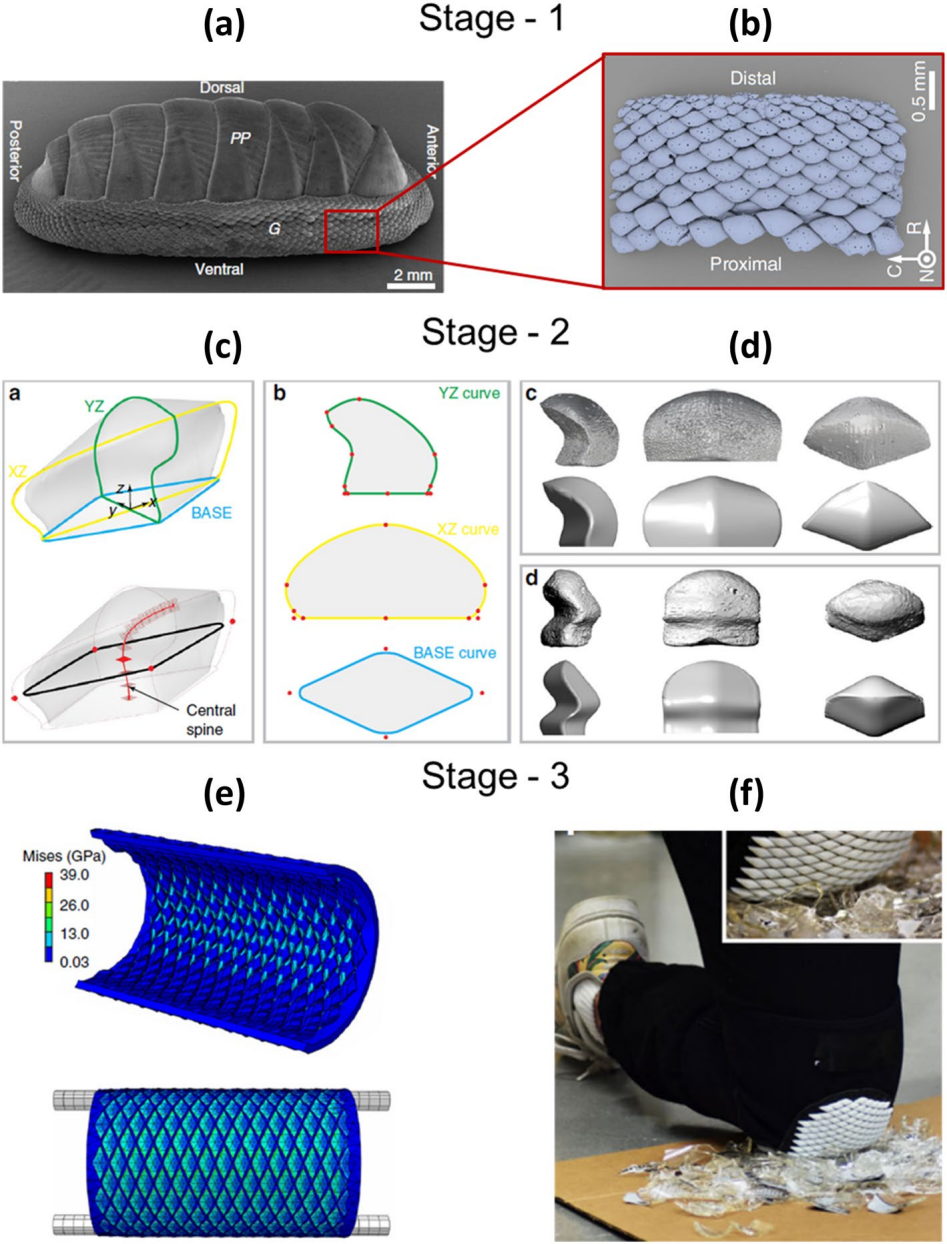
a SEM image of chiton’s armour and b segmented X-ray volume; c sketches of parametric CAD model; d comparison between the CT data-based CAD design and the segmented 3D volume of the actual chiton armour components; e virtually buckled FEA model of man-made armour; f a prototype of chiton-inspired armour (reproduced from [313])
